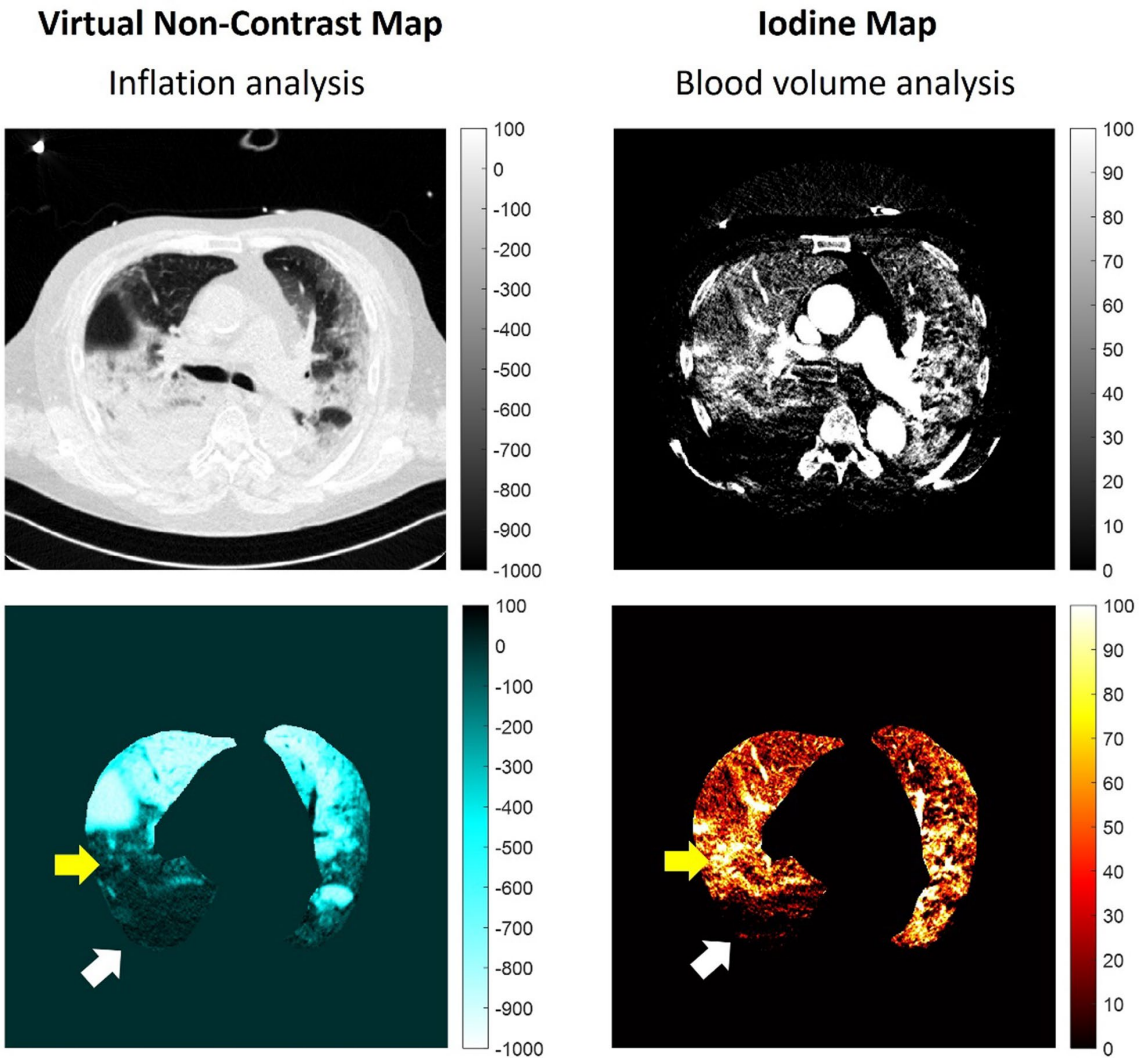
DECT COVID-19 ARDS patient. A representative example of a reconstructed image at the carina level, part of a static chest DECT scan. Left: virtual non-contrast (VNC), no-contrast agent CT reconstruction, used for inflation/collapse analysis (as a surrogate index of ventilation). Below: colormap highlighting non-inflated regions (-100 to +100 HU) in black and hyperinflated regions (-900 to -1000 HU) in white. Right: iodine map reconstruction, used for blood distribution analysis (as a surrogate index of perfusion). Below: colourmap highlighting perfused regions (0 to +100 HU) in black and not perfused areas (lower or equal to 0 HU). In this specific case, there are both inflation and perfusion defects, mainly represented in the dorsal region of the left lung (white arrows). At the same time, a hyperperfused region corresponds to a hypo/not inflated lung region in the middle portion of the left lung (yellow arrows). Clinical data: 90kg, male, 68 y. NIV (PSV) P_insp_ 2 cmH_2_O, PEEP 6 cmH_2_O. Vt/PBW = 8.9 mL/kg, RR = 19/min. ICU stay 3 days. Discharged alive.
